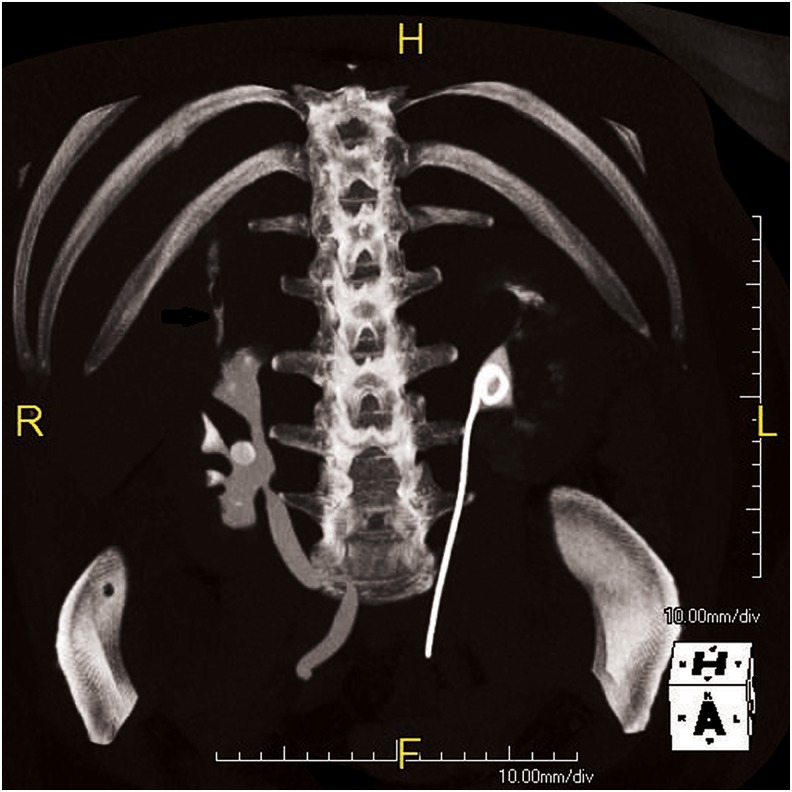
Axial cut of CT abdomen/pelvis with *arrow* showing fistula. CT, computed tomography.
